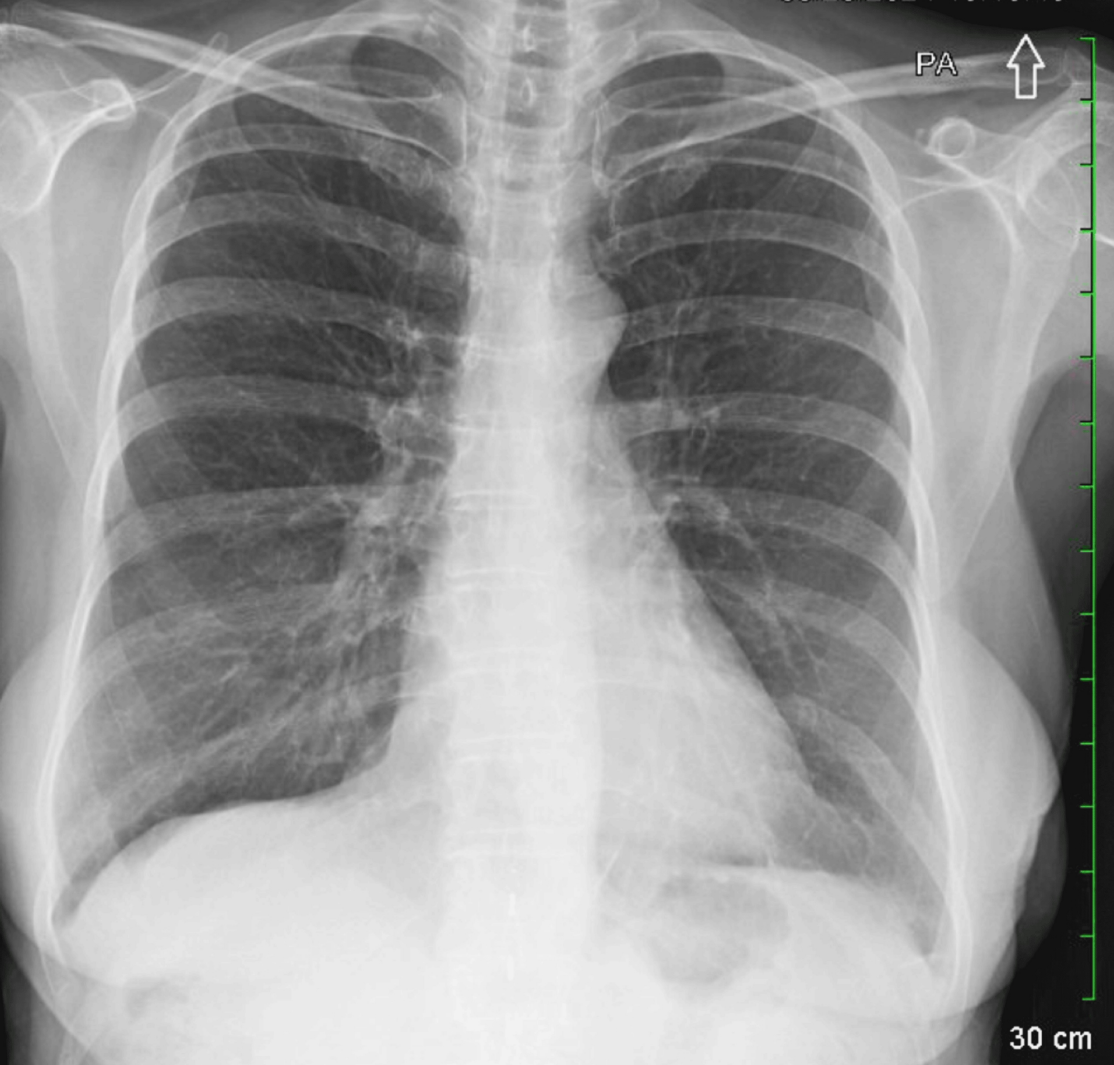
Repeat chest radiograph at discharge showing no evidence of PM PM, pneumomediastinum
